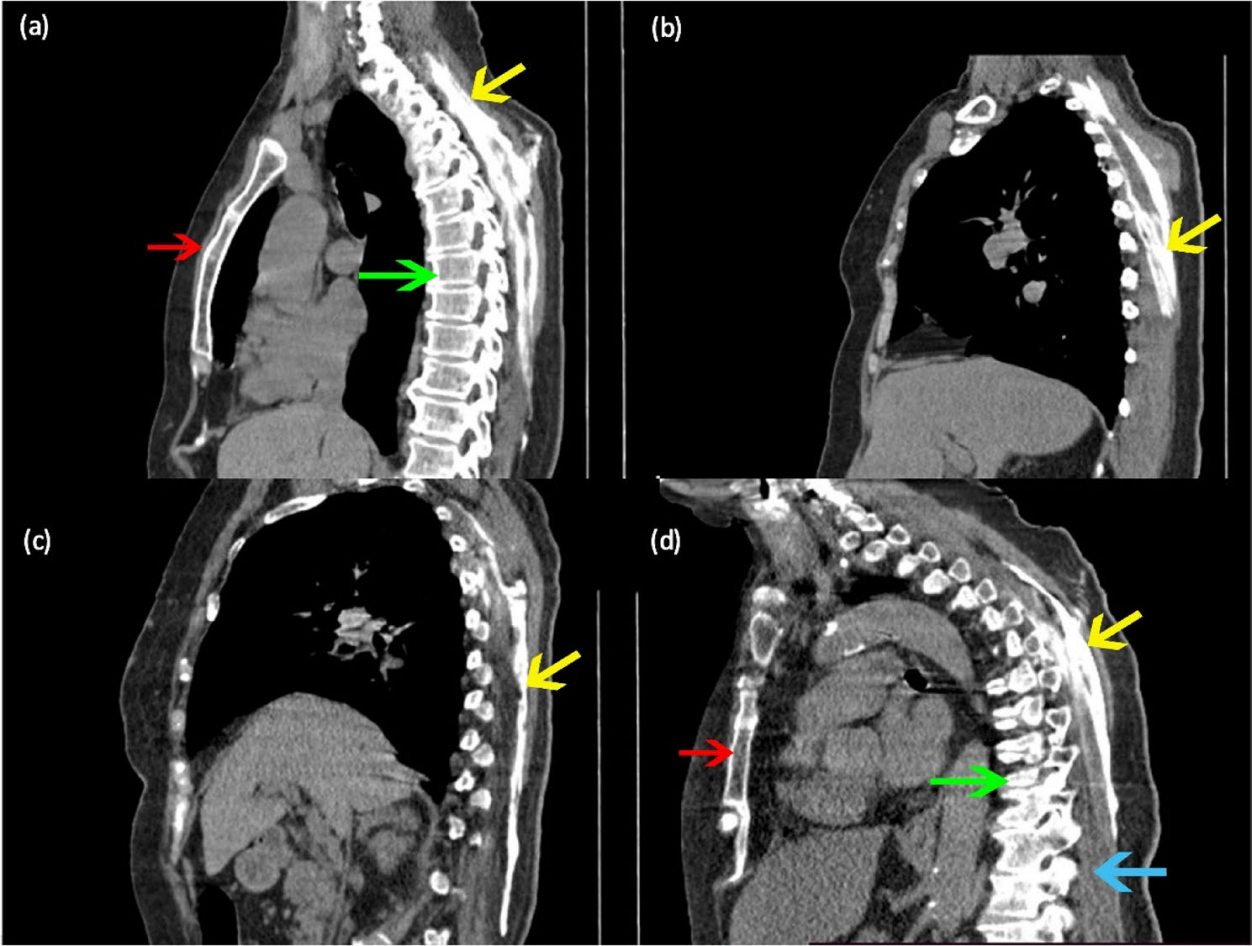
Different sagittal computed tomography cuts (a-d) of the thoracic region in different patients show retained contrast (yellow arrows) along the erector spinae muscle. Cuts (a, d) show the manubrium sterni (red arrows), cuts (a, d) show the dorsal spine (green arrows), and Cut (d) shows the erector spinae muscle (blue arrow)
